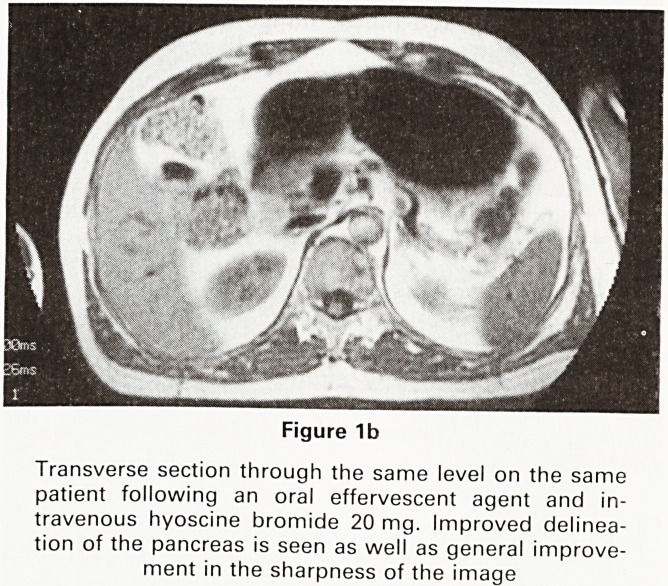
# Magnetic Resonance Imaging of the Pancreas
